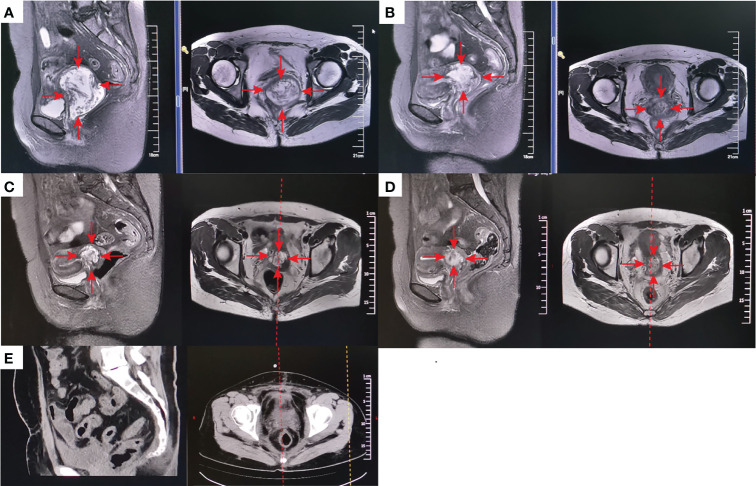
A patient with stage IB3 at the initial diagnosis of mucinous adenocarcinoma of the cervix developed into a huge residual mass of 57^*^76^*^66mm (MRI) (A) because of the treatment was terminated by grade 4 myelosuppression due to one cycle of neoadjuvant chemotherapy, which also made the patient refuse concurrent chemotherapy. After external-beam radiotherapy (EBRT) 30Gy plus 1 cycle local injection of H101 (1.0×10^12^ virus particles daily for 5 days), the mass was significantly reduced to 46^*^51^*^48mm (MRI) (B). The mass was further reduced by 32^*^46^*^45mm (MRI) (C) after 50Gy of EBRT. After the following brachytherapy (6Gy for 1 time of intraluminal brachytherapy and 7Gy for 3 times of interstitial brachytherapy), the residual lesion was 29^*^39^*^30mm (MRI) after 1 month of radiation (D). Since refusal of chemotherapy, she accepted 1 cycle treatment of H101 (5.0×10^11^ virus particles daily for 5 days) again. Gynecological examination indicated that the lesion had further shrunk, while there were still some suspicious and hard masses. Therefore, surgical resection of the residual lesion was suggested. Postoperative pathology indicated that no cancer cells were found in the surgically excised lesions. One-month postoperative Computed tomography (CT) scan showed no signs of tumor (E). The red arrow stands for the tumor mass.
